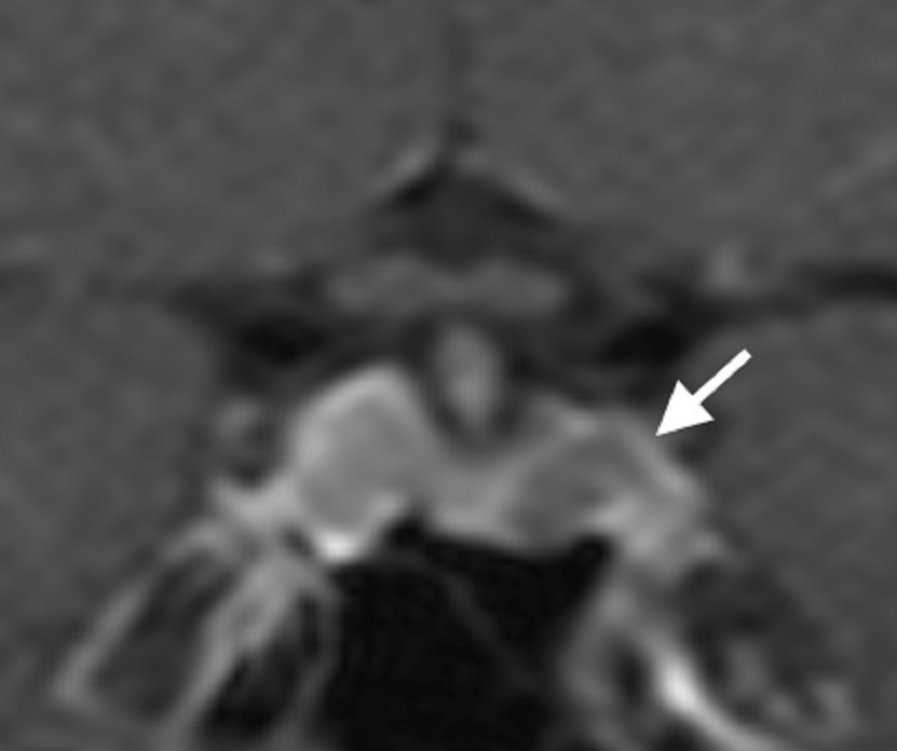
Coronal Post-Contrast MRI Sequences Following initial successful treatment with bromocriptine (arrow shows tumor within gland)
